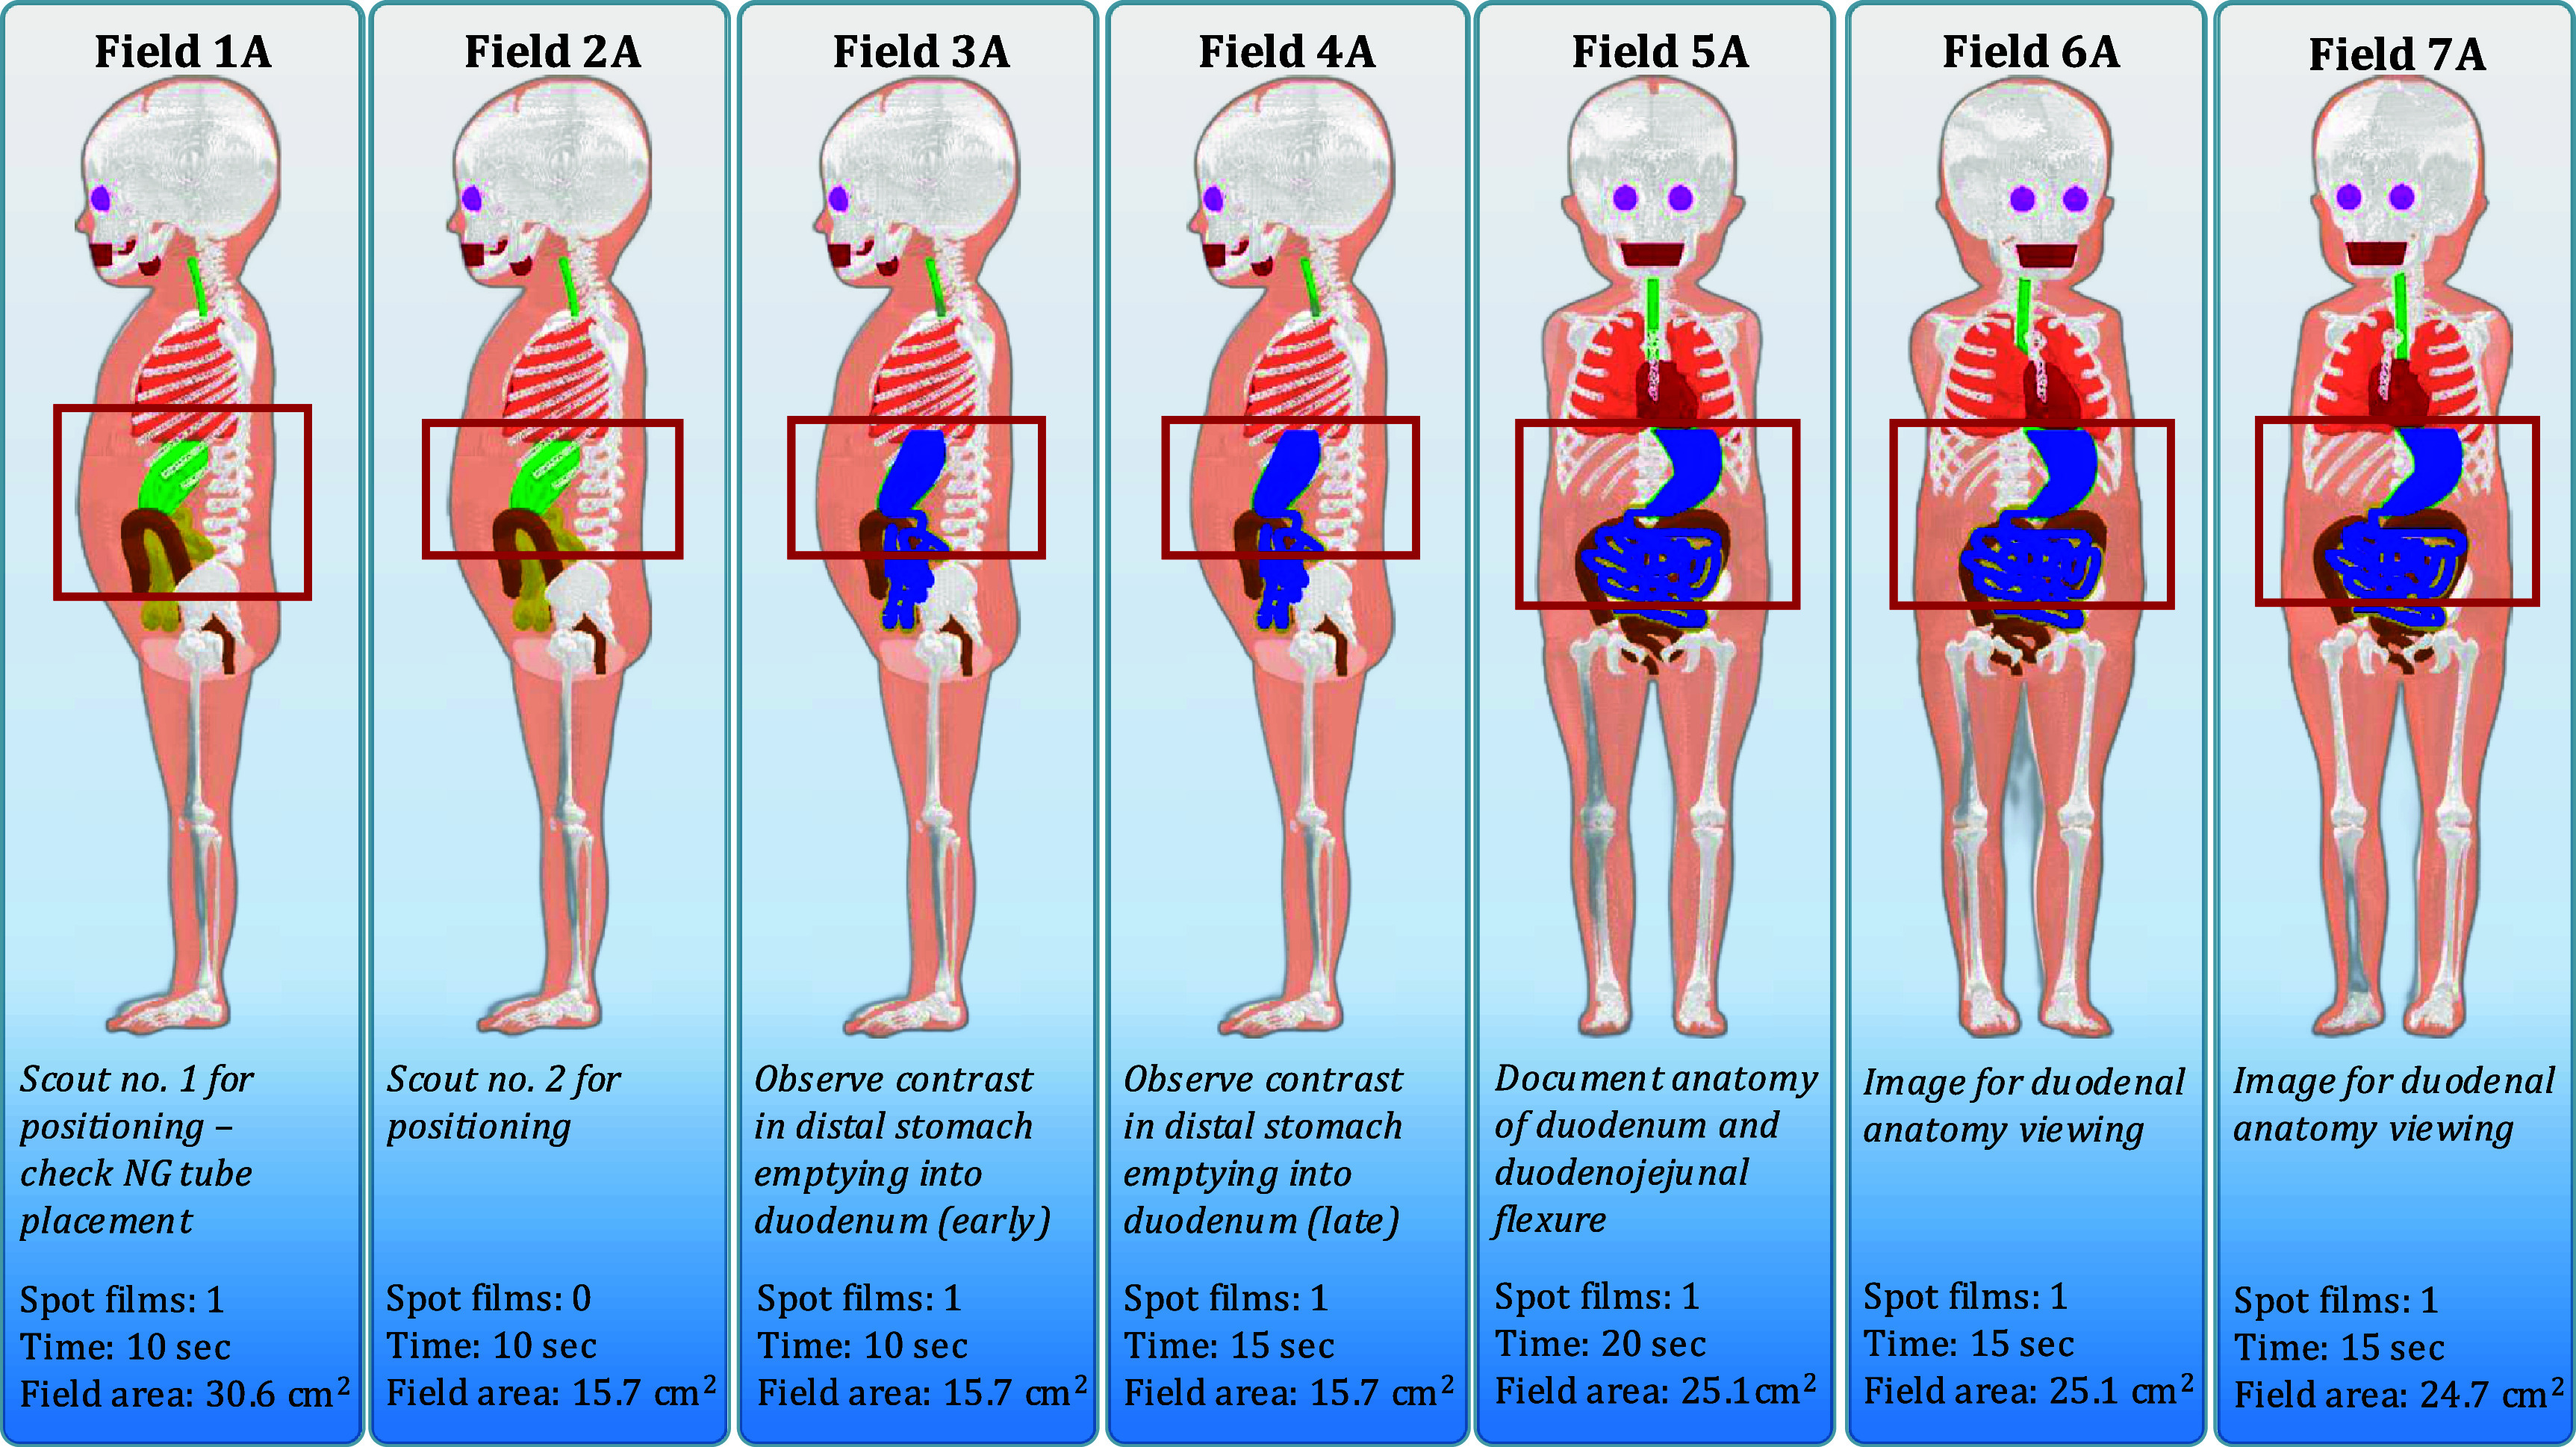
Examination outline with demarcated fields for a 1 year-old female undergoing a UGI series resulting in an abnormal diagnosis. Field area calculated at 20 cm from the x-ray source.
